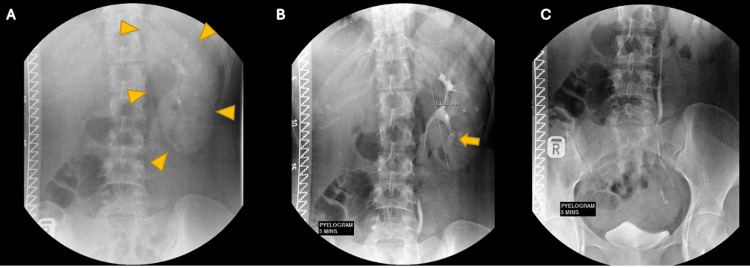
IVU findings IVU in the immediate nephrogenic film (A) shows an absence of the right kidney nephrogram with a normal left renal nephrogram (yellow arrowheads). (B-C) Five-minute pyelographic films show the absence of the right renal collecting system. There is a filling defect in the left lower pole collecting system consistent with the renal calculus, as seen in the ultrasound (yellow arrow) IVU: intravenous urogram
